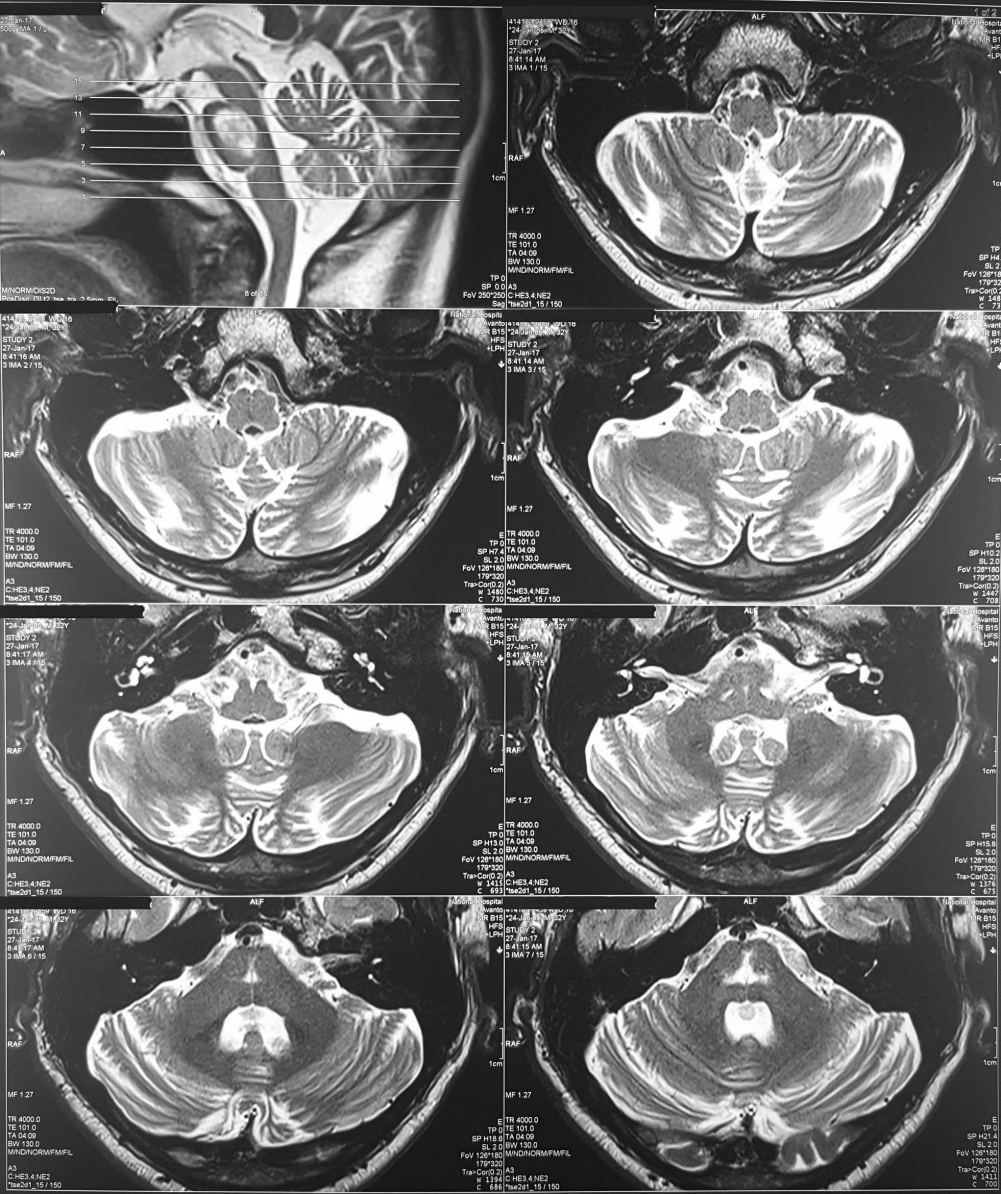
MRI of the brain stem, done 1 month after the first MRI showing normal medulla and hypoglossal nuclei
